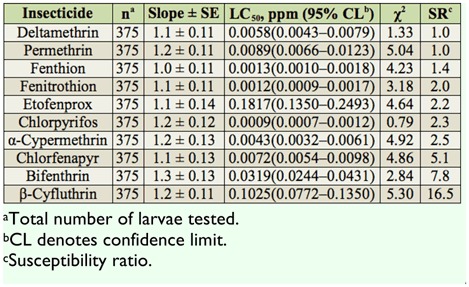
Summary of toxicities of 10 insecticides to third instars of the CC-CT strain of *Culex tritaeniorhynchus* based on the 24 hr exposure contact mortality bioassays.
